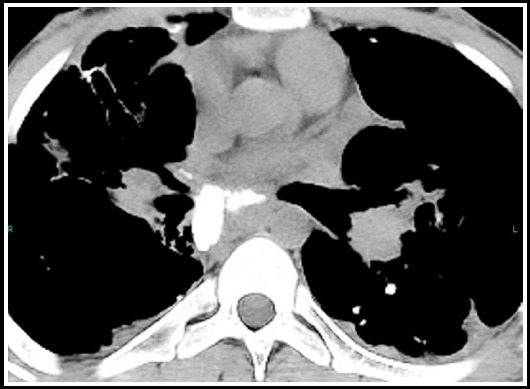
CT scan section in mediastinal window setting, shows calcified mediastinal lymph nodes.
